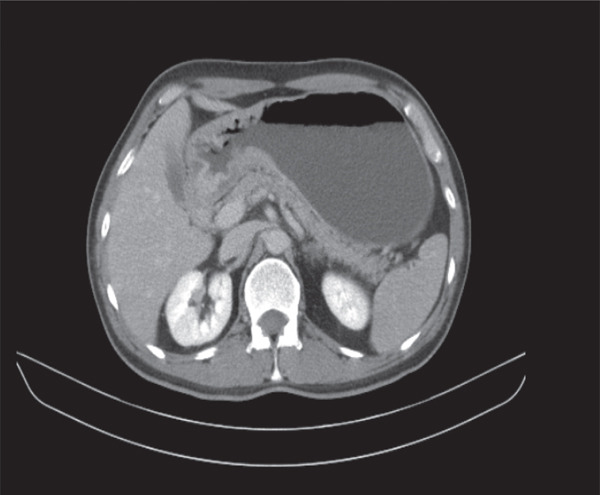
Diagnostic CT showing diffuse thickening of the duodenal and jejunal walls.
